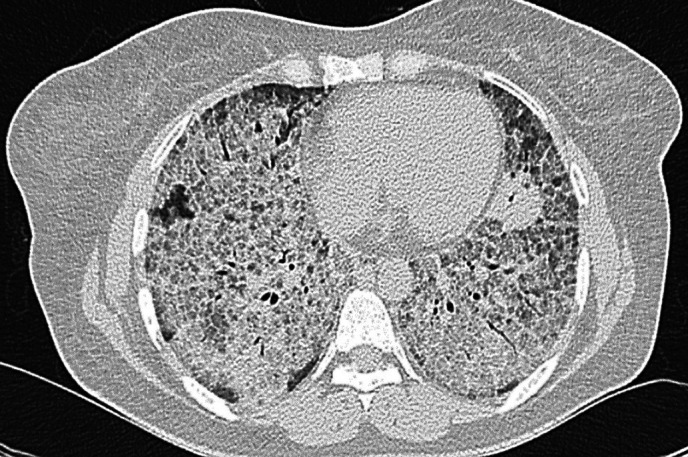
High-resolution computed tomography chest of Case 1, showing bilaterally diffuse ground-glass haziness with a network of smooth, interlobar septal thickening, with a characteristic “crazy-paving” appearance.
